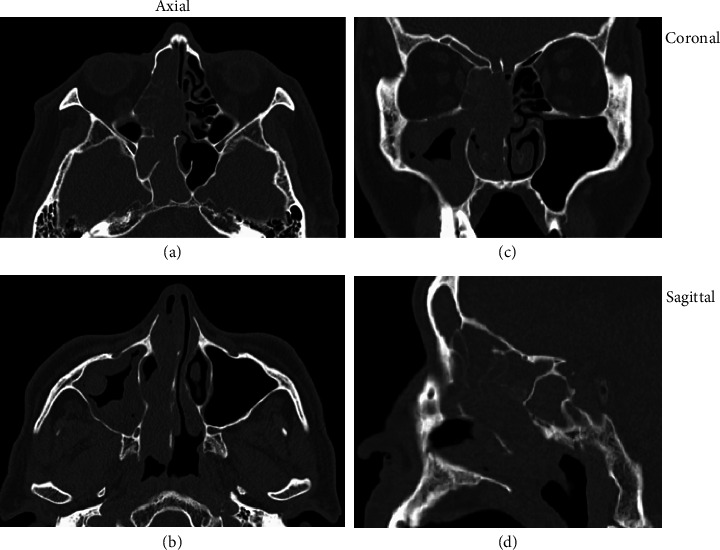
Computed tomography findings. (a, b) Axial CT images show a soft tissue mass occupying the right paranasal sinuses. Image (a) is taken at the level of the sphenoid sinus, whereas image (b) is taken at the level of the maxillary sinus. The nasal septum is deviated to the left. (c) Coronal CT view and (d) sagittal CT view show no evidence of bone destruction or orbital invasion.
